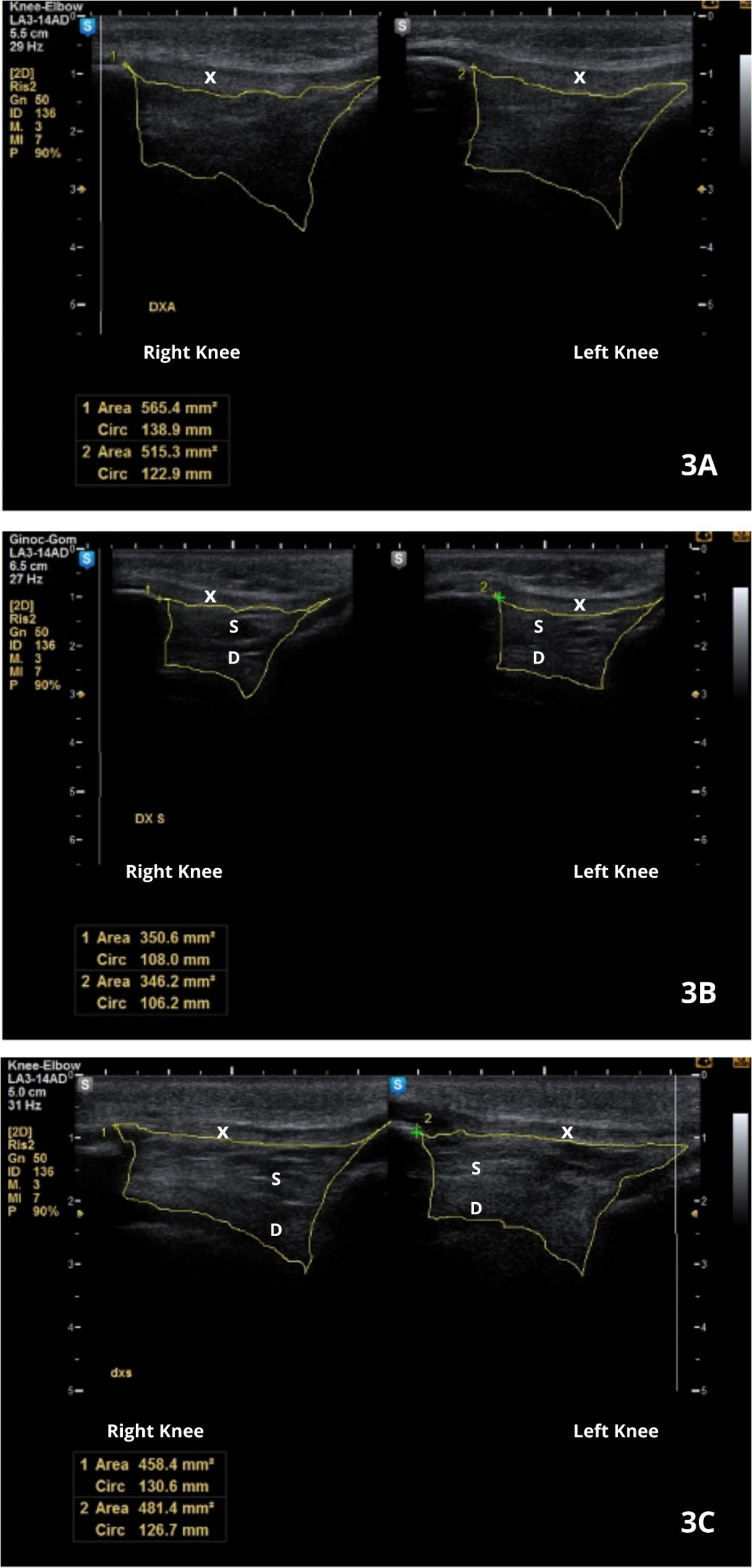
US evaluation of Hoffa’s fat pad. 3 A-3 C B-mode US images of the knee on the longitudinal subpatellar scan plane, which provide an evaluation of Hoffa’s fat pad (area outlined in yellow) echostructure and echogenicity, compared to the patellar tendon (X). All patients were 9-year-old prepubescent females. 3 A Patient with higher BMI (BMI = 29.31, > 99th percentile) presents with hypoechoic fat pads with unidentifiable double-layer structures and larger fat pad areas (565.4 mm² right, 515.3 mm² left), potentially indicating increased tissue volume and altered composition due to greater mechanical or inflammatory stress. 3B,3 C Patients with lower BMIs (respectively BMI = 23.13, 95–99th percentile, 3B; BMI = 21.41, 85–95th percentile, 3 C) presented slightly hypoechoic fat pads with identifiable superficial septate (S) and deep homogeneously hypoechoic (D) layer structures and smaller fat pad areas (350.6 mm² right, 346.2 mm² left for BMI 23.13), which may reflect reduced mechanical stress and more preserved tissue characteristics. The OMERACT US semiquantitative scoring system revealed no signs of inflammation, such as effusion or hypervascularization, in any of these patients. Abbreviations: BMI, body mass index; OMERACT, Outcome Measures in Rheumatology; US, ultrasound
